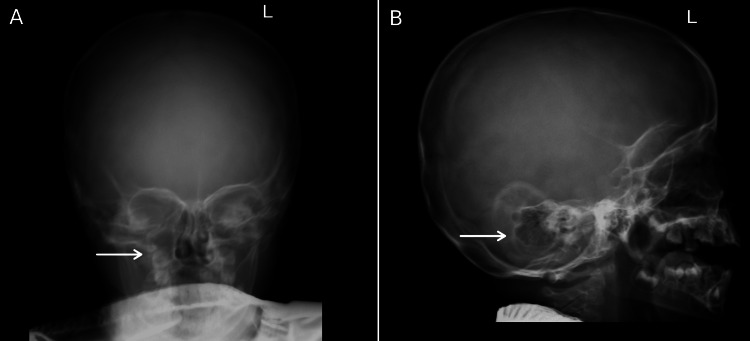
X-rays of the skull showing multiple oval-shaped destructive foci in the bones with unclear contours.
